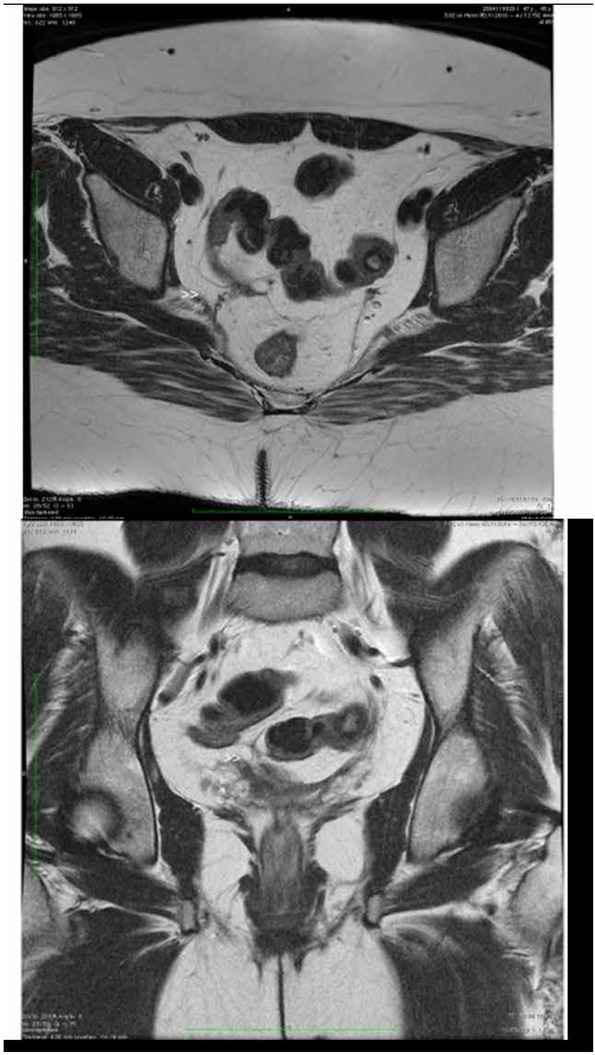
Abdomen- Pelvic MRI . Fibrotic abnormalities, with prevalence lamellar, that branch off on the right from the vaginal hemicupole, from which they reach the right ureter in the pre-bladder tract adhering to the posterior wall of the sigma 1 A - Axial T2 weighted FSE 4mm 1 B - Coronal T2 weighted FSE 4mm
